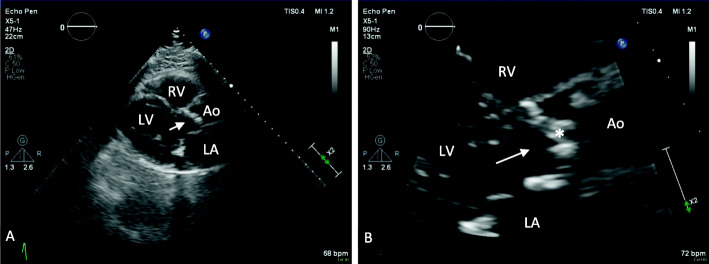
Trans-thoracic parasternal long-axis echocardiographic view. a This example demonstrates a hypertrophic left ventricle (LV) and calcified aortic valve (arrow in the left ventricular outflow tract pointing toward aortic valve. b This example demonstrates a severely calcified aortic valve (*)
